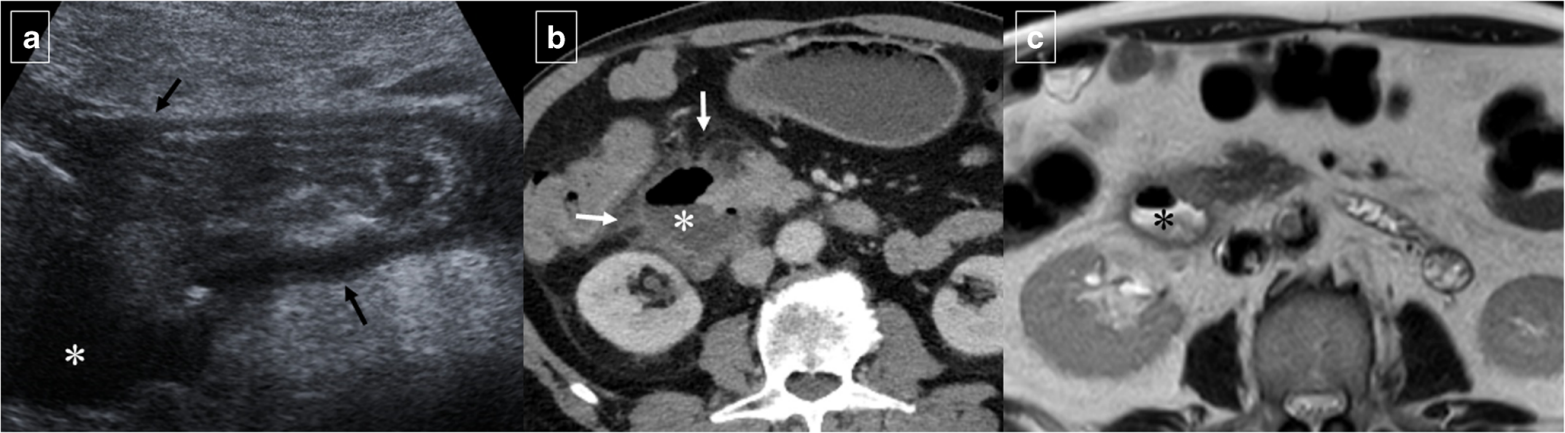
A 67-year-old man with epigastralgia, fever, and food intolerance. a Initial abdominal ultrasonography shows diffuse thickening of the different layers of the duodenal wall with a slight amount of free periduodenal fluid (arrows) and an adjacent hypoechoic fluid collection (*). b Axial contrast-enhanced CT confirms the presence of a paraduodenal collection with an air-fluid level (*) and surrounding soft-tissue stranding (arrows) caused by acute duodenal diverticulitis. c Axial T2-weighted MRI after conservative therapy shows the outpouching from the duodenal wall is smaller and more well-defined; it now has the appearance of a duodenal diverticulum, and the amount of soft-tissue stranding has decreased
